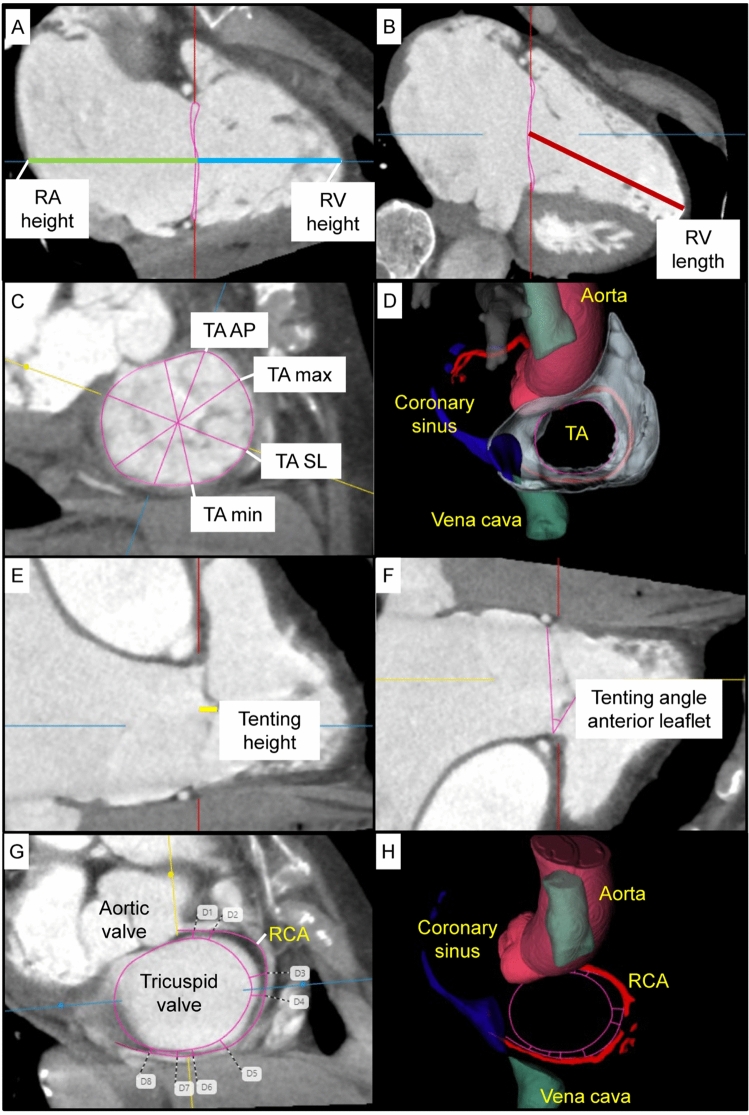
Computed tomography (CT) analysis of right atrial (RA) and right ventricular (RV) height (A), RV length (B), tricuspid annulus (TA) with antero-posterior (AP), septo-lateral (SL), minimal (min) and maximal (max) diameter (C, D), tenting height (E), tenting angle of the anterior leaflet (F), and distance between tricuspid annulus and right coronary artery (RCA, G, H).
